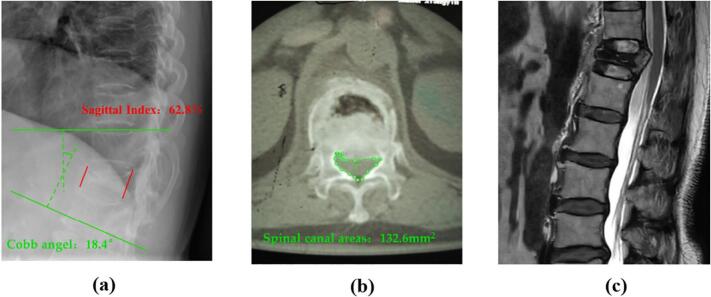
Preoperative lateral X-ray, sagittal MRI and transverse CT were performed.
